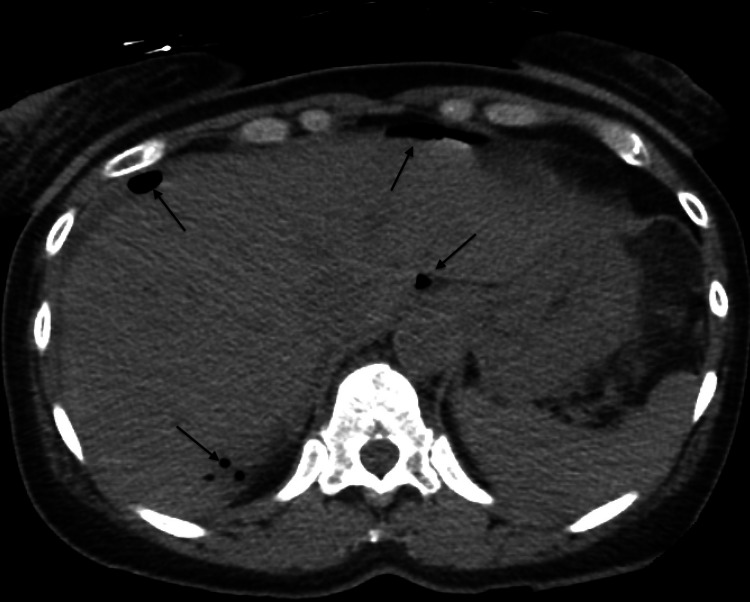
computed tomography axial image Pneumoperitoneum (arrows)
